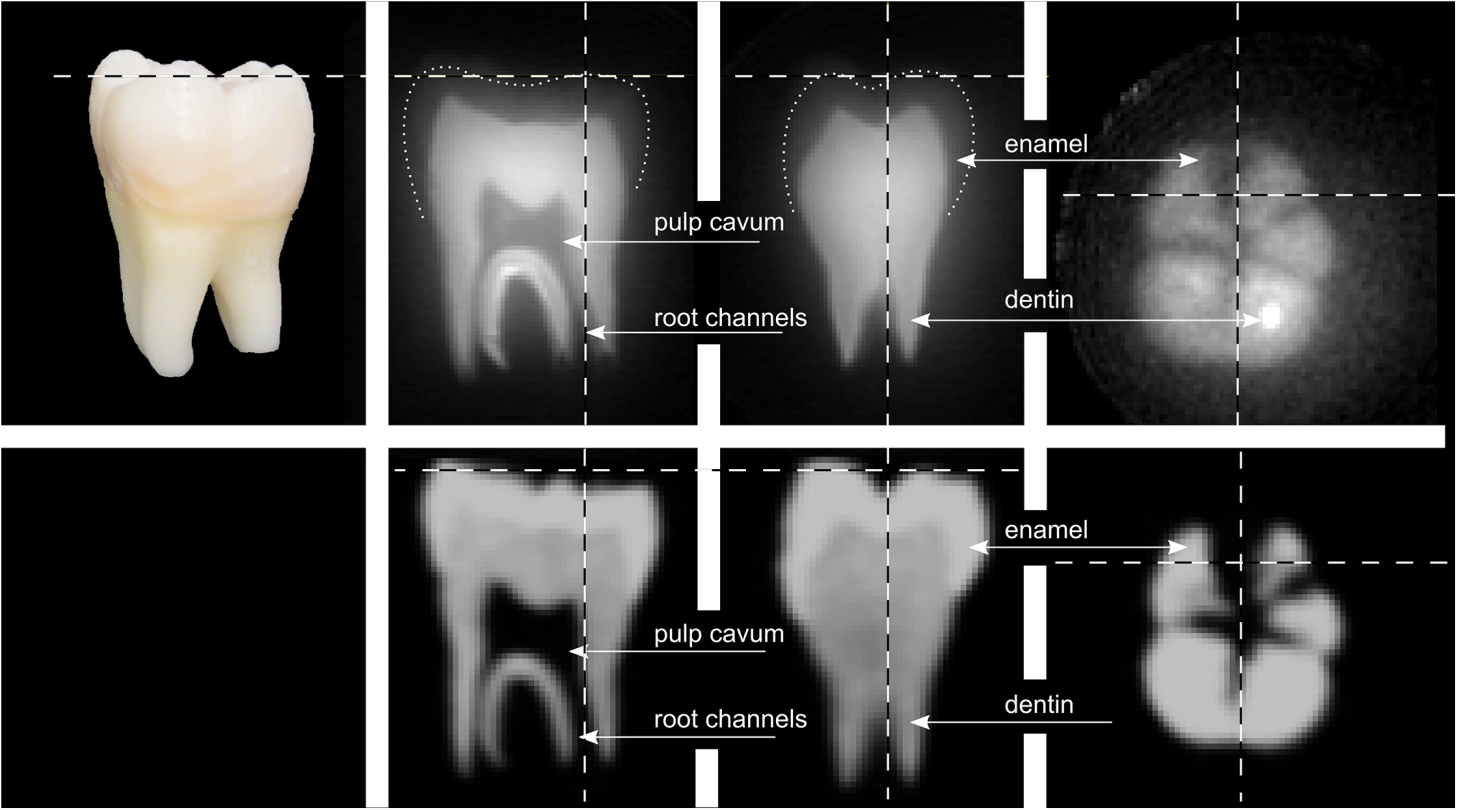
Photograph (top left), orthogonal reconstructions of a 3D ZTE MRI of an extracted human molar acquired at 9.4 T with CB_2_ and LG_1_ (top row) and 3D CBCT (bottom row). Note that the brightness of the MRI on the right was adjusted to show the enamel. Dashed lines indicate the slice positions, dotted lines the approximate outline of the enamel.
